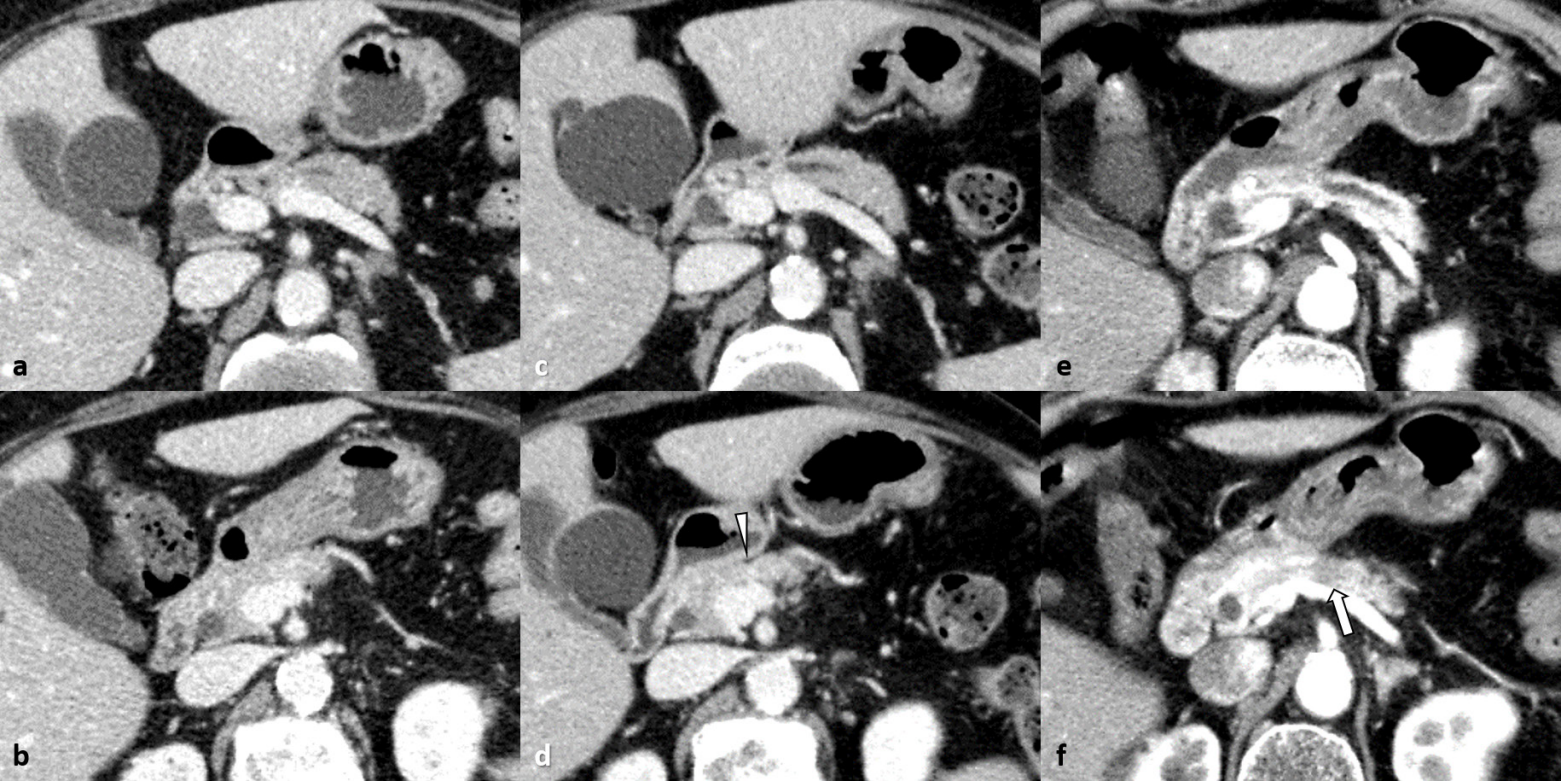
An 86-year-old female with pancreatic adenocarcinoma that developed after treatment for malignant melanoma of the left lower limb (Case 5) (a, b). Venous phase contrast-enhanced CT image obtained 24 months before the pancreatic surgery showing no abnormal findings suggestive of pancreatic adenocarcinoma. (c, d) Contrast-enhanced CT images obtained 15 months before the pancreatic surgery showing mild dilatation of the pancreatic duct. There is partial parenchymal atrophy (arrowhead) corresponding to the location of pancreatic duct interruption. (e, f) Triple-phase contrast-enhanced pancreatic phase CT image obtained immediately before pancreatic surgery showing a small hypoattenuated pancreatic body mass (10 mm diameter, arrow) and obvious upstream pancreatic duct dilatation. The pancreatic duct is interrupted by the mass, which is invading the peripancreatic fat tissue.
